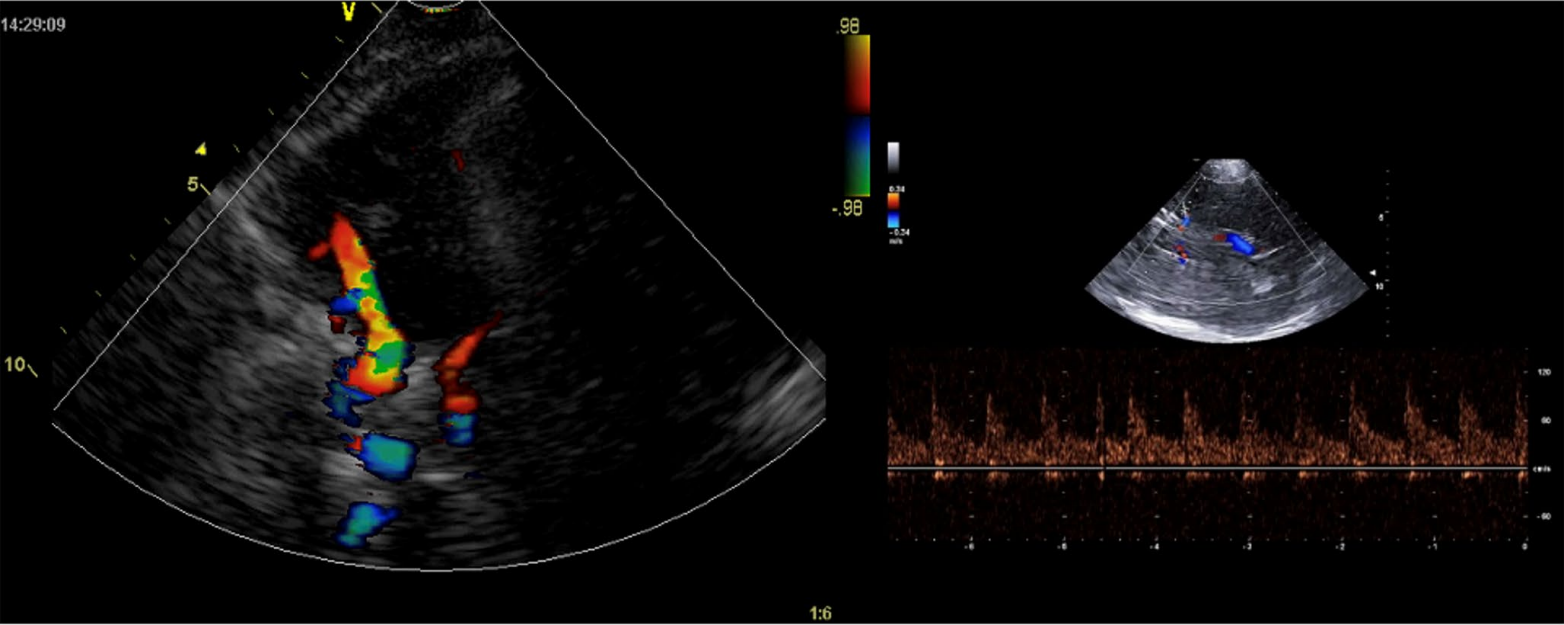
TCCD, color and B-mode. TCCD combines B-mode with Doppler pulse wave technology. The B-mode imaging shows an image of the skull, brain, and blood vessels. Once the desired blood vessel is found, it is shown with the color function
